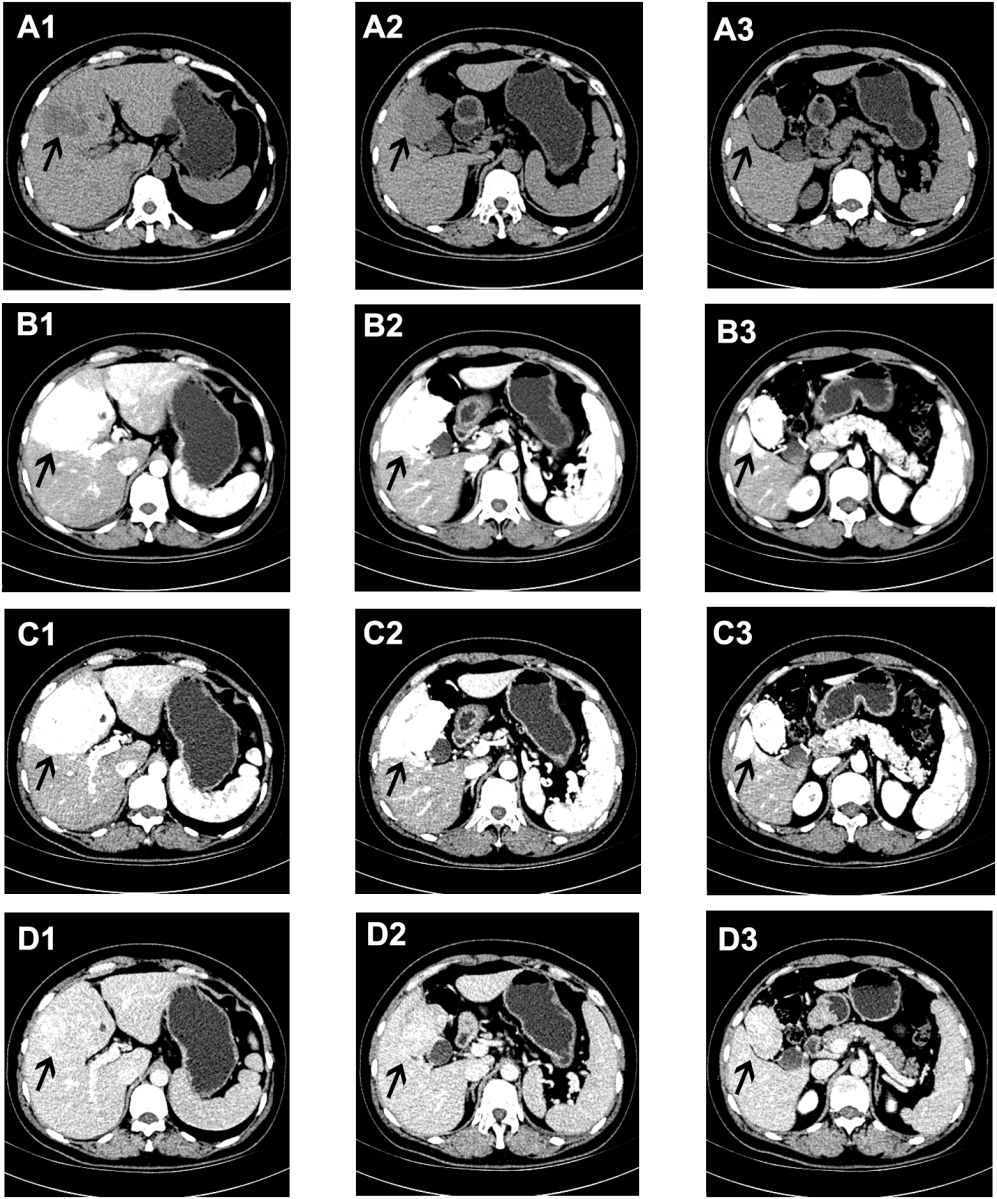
Abdominal enhanced CT manifestations of the lesions in the liver. (A1–3) On a plain CT scan, a poorly defined low-density lesion could be seen in the S4b and S5 segments of the liver. In the arterial phase (B1–3) and venous phase (C1–3) of a contrast-enhanced CT scan, the lesions showed continuous and obvious enhancement, while in the delayed phase (D1–3) the enhancement of the lesions decreased, and the density was similar to that of the normal liver, as shown by the black arrow.
